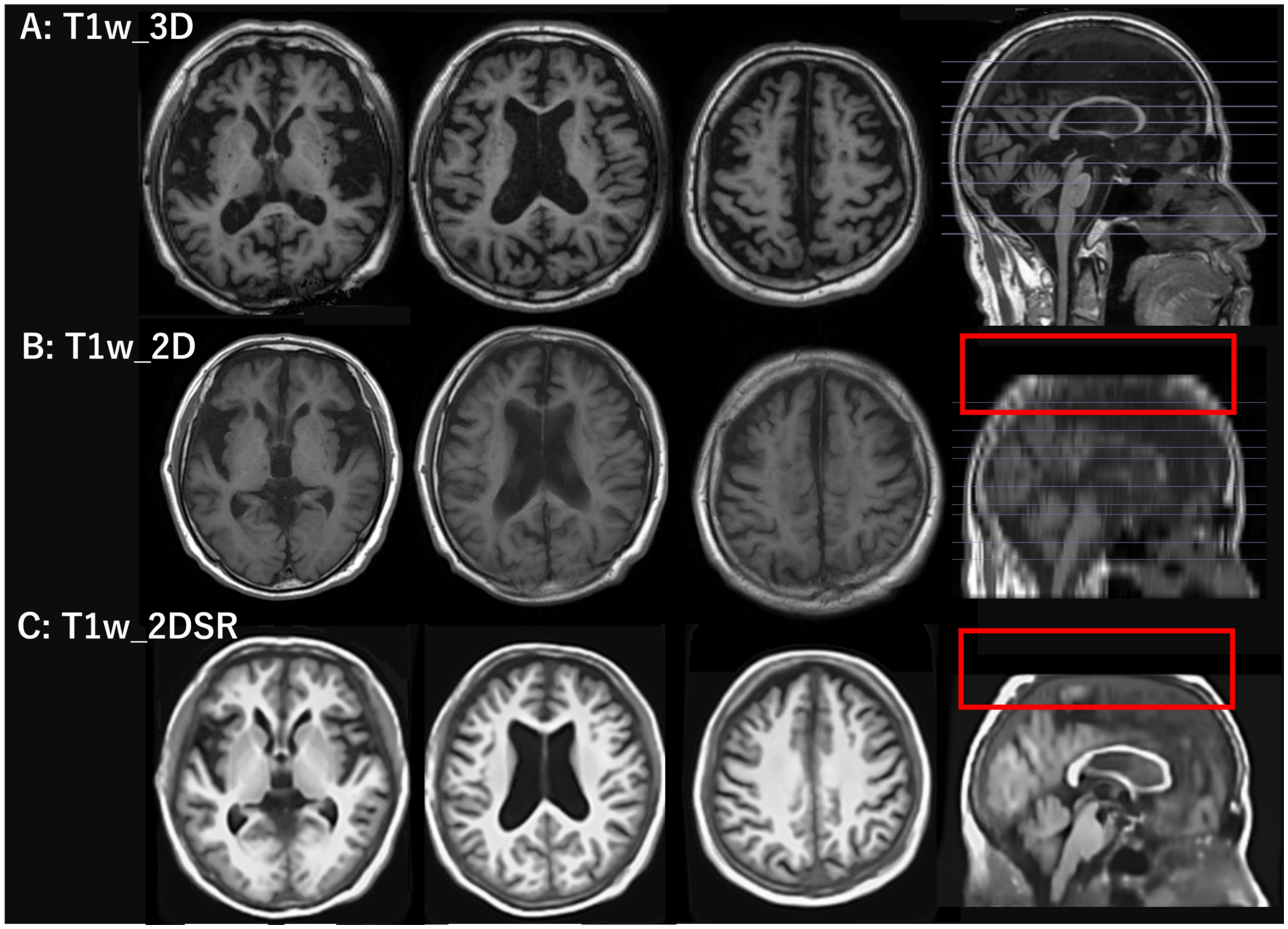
T1-weighted images used in the standard and proposed methods. The top row shows 3D T1-weighted images acquired using spoiled gradient recalled acquisition in steady state (SPGR) sequence with the standard method. The middle row shows 2D T1-weighted images acquired using periodically rotated overlapping parallel lines (PROPELLER), which are commonly used in clinical settings. The bottom row shows 3D volume data generated from 2D T1-weighted images using FreeSurfer’s SynthSR tool. The red rectangles in the middle and bottom rows indicate that the parietal region was not included in the original scan and therefore could not be generated.
